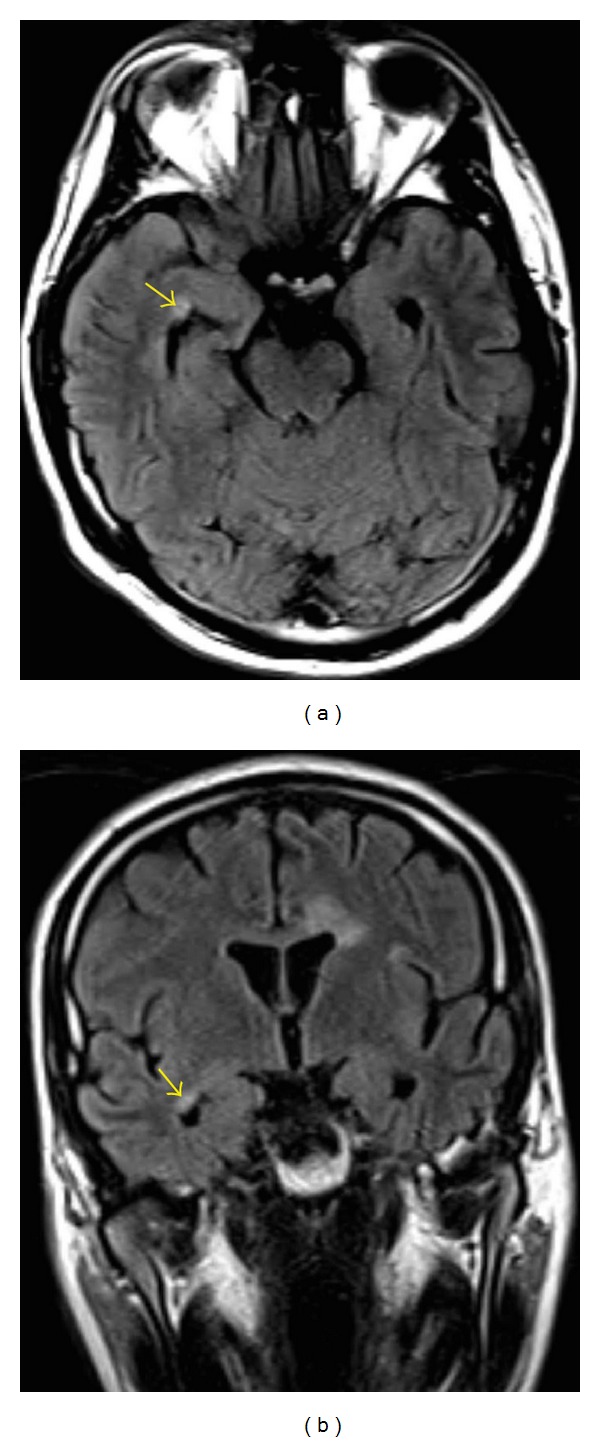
Follow-up cranial MRI showing (a) axial and (b) coronal T2-weighted FLAIR view of the new demyelinating lesion in the right temporal white matter (arrows).
